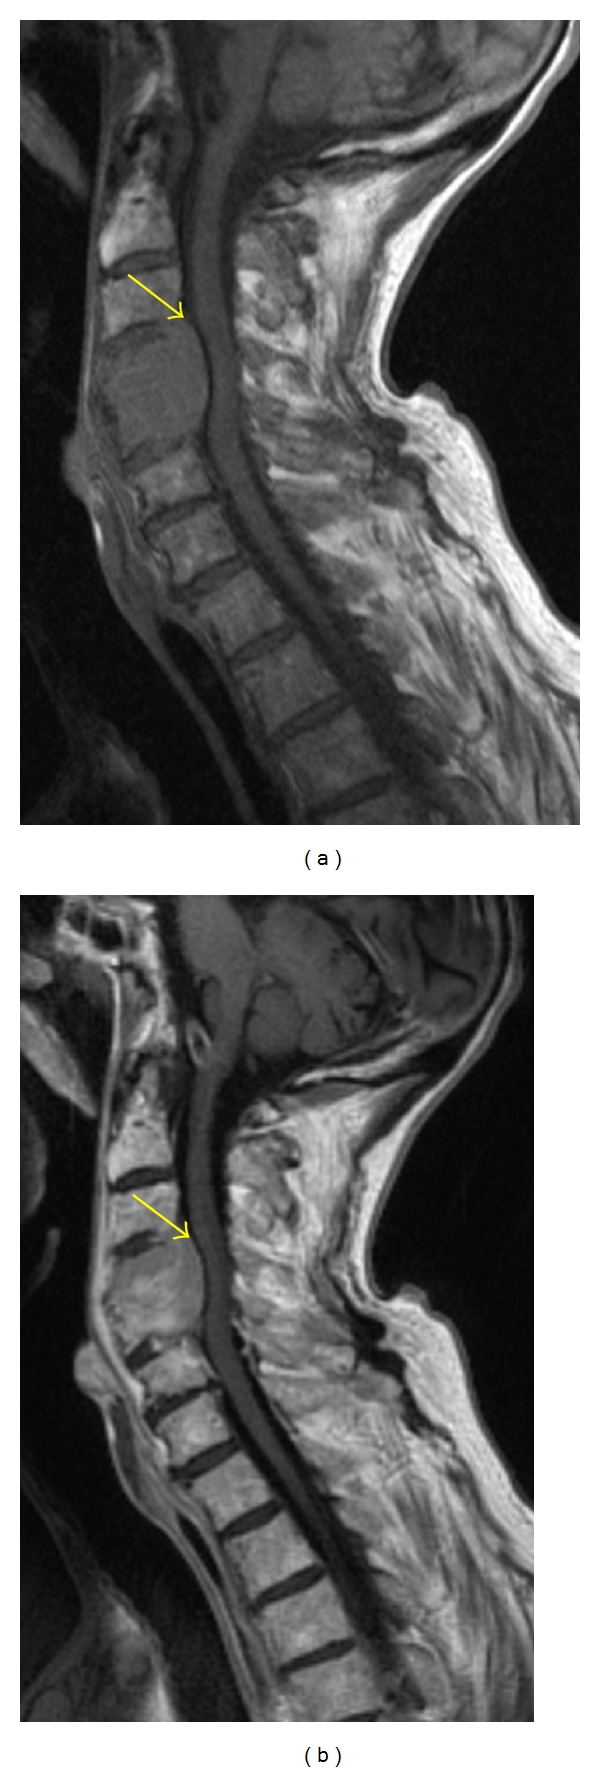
Sagittal T1-weighted MR image (a) shows a hypointense expansile lesion involving the C4 and C5 vertebral bodies with extension into the ventral epidural space (yellow arrow). The lesion enhances homogeneously on postcontrast T1-weighted MR (b); however, the degree of normal marrow enhancement is similar to that of the metastatic myeloma lesion.
